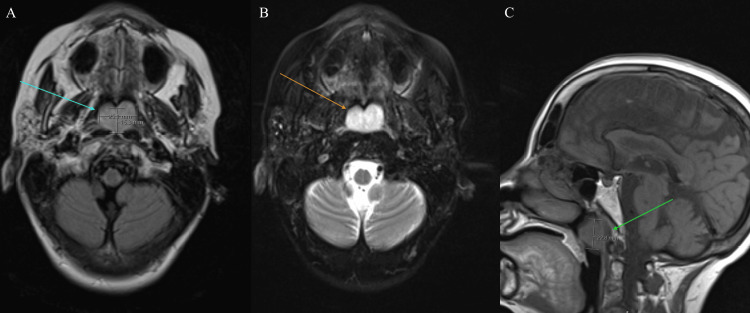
A prominent lobulated lesion measuring approximately 2.5 x 1.6 cm in cross-sectional dimension (A) within the nasopharynx, originating from the posterior pharyngeal wall, demonstrated hyperintense signals on FLAIR imaging and intense postcontrast enhancement (B). It measured 2.3 cm in craniocaudal dimension (C). FLAIR, fluid-attenuated inversion recovery
